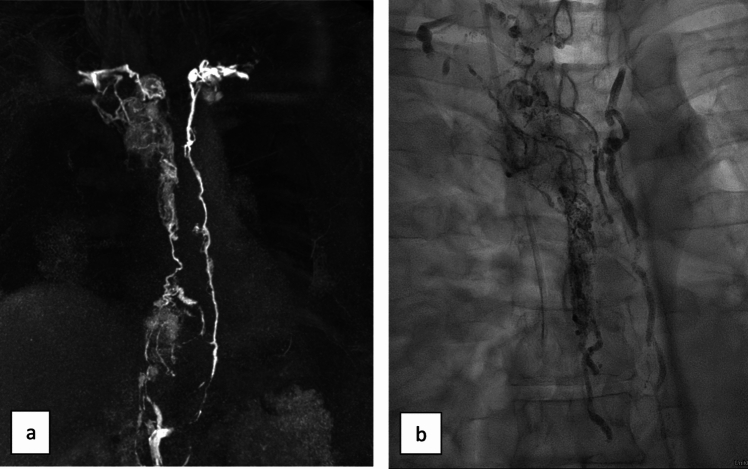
MR (a) and X-ray lymphangiography (b) of a 52-year-old patient with DLBCL and refractory non-traumatic chylothorax. There is a marked thoracic lymphatic flow disturbance with significant thoracic duct obstruction in the upper part and chylolymphatic reflux into alternative pathways in the middle and upper thirds of the thorax. Lymphatic enhancement was first visible in the alternative pathways on the right and only secondarily and delayed of the thoracic duct
